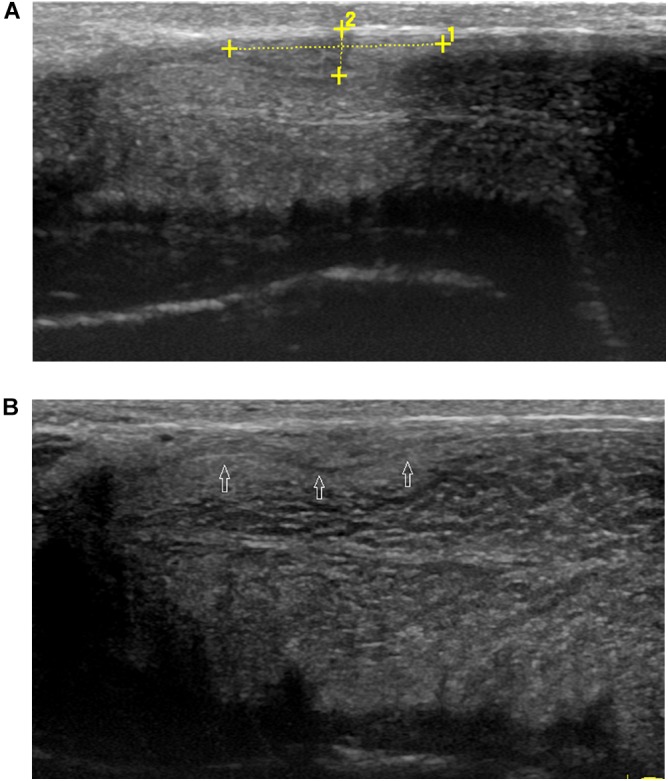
Palpable ventral plaque of left corpus cavernosum. (A) Longitudinal sonogram, oblique ventral access (from the midline to the left lateral face of the penis). Fuzzy ventral tunica thickening of left corpus cavernosum in the flaccid penis. (B) Longitudinal sonogram, oblique ventral access (from the midline to the left lateral face of the penis). With the corpora cavernosa expansion, the local tunica albuginea thickening becomes obvious (arrows) in the erect penis.
